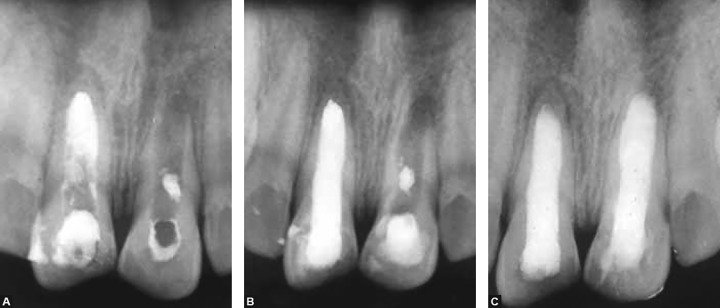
(A) MTA apical plug in tooth 11, (B) after gutta-percha filling of remaining canal in tooth 11 next day and MTA apical filling in tooth 21, (C) final radiograph after gutta-percha filling in tooth 21
